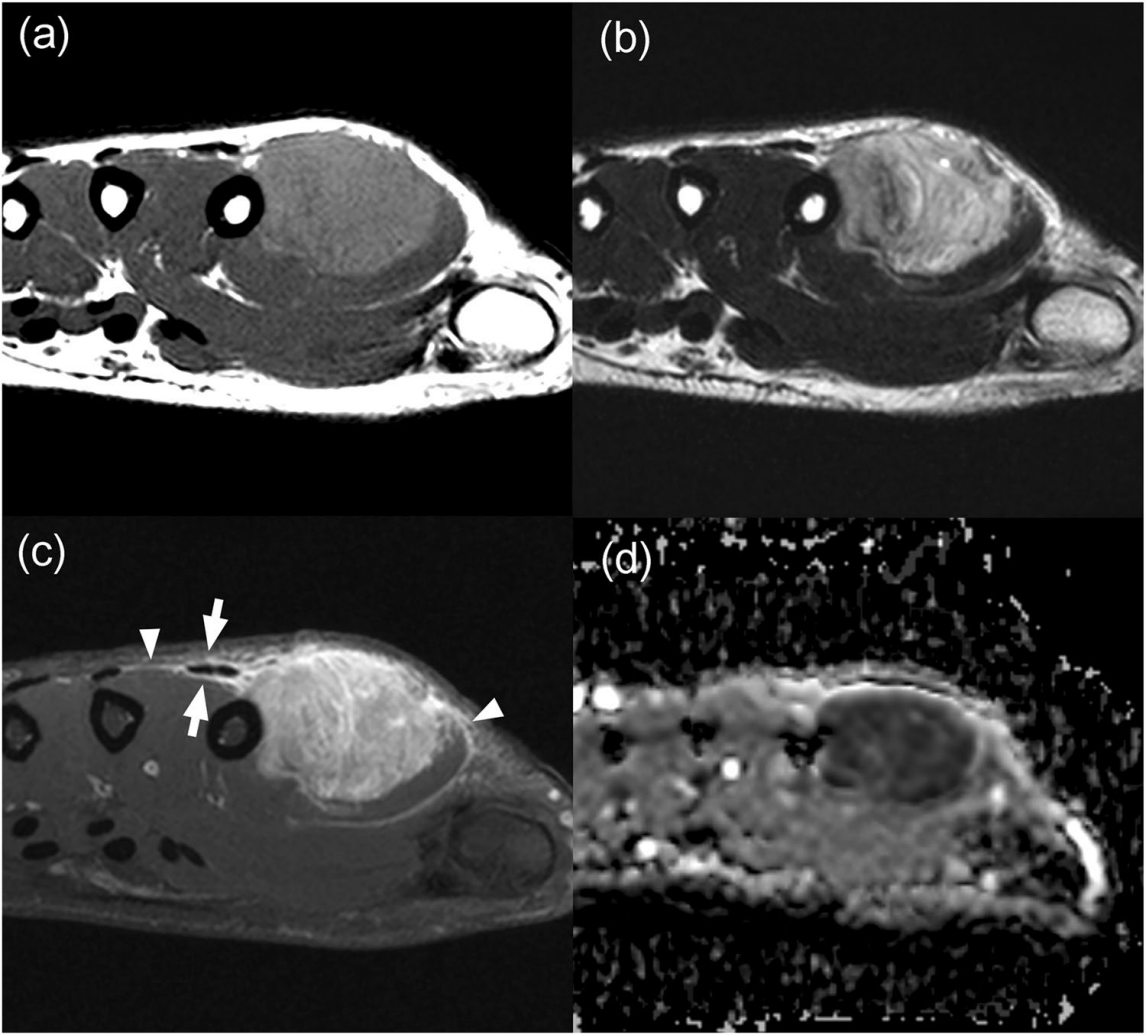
A 20-year-old man with alveolar rhabdomyosarcoma. (a) Axial T1- and (b) T2-weighted images of the left hand showing a lobulated mass with mean size of 2.9 cm and peritumoral edema (not shown). Split fat sign was not evident. (c) Heterogeneous enhancement was seen on the axial fat-suppressed contrast-enhanced T1-weighted image. Infiltration along the extensor tendon (arrows) and tail sign (arrowheads) were noted. (d) The mean and minimum ADC values of the lesion were measured to be 0.85 × 10^−3^ mm^2^/s and 0.46 × 10^−3^ mm^2^/s, respectively. The probability of malignancy was calculated to be between 0.8–0.9 by both models I and II.
